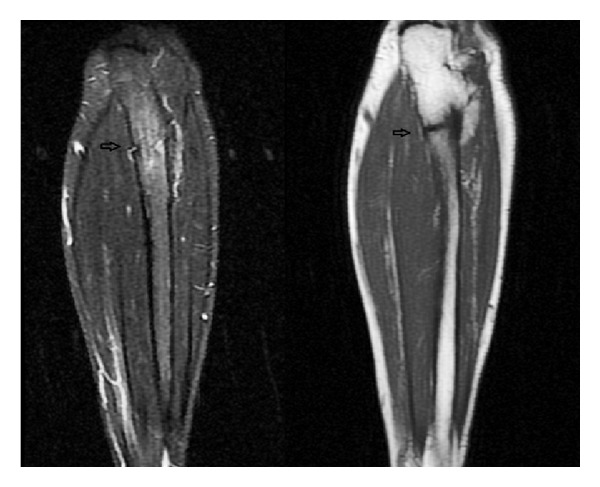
Coronal sections showing stress fracture line and surrounding soft tissue oedema in T1 and T2 sequences of the patient's forearm MRI.
